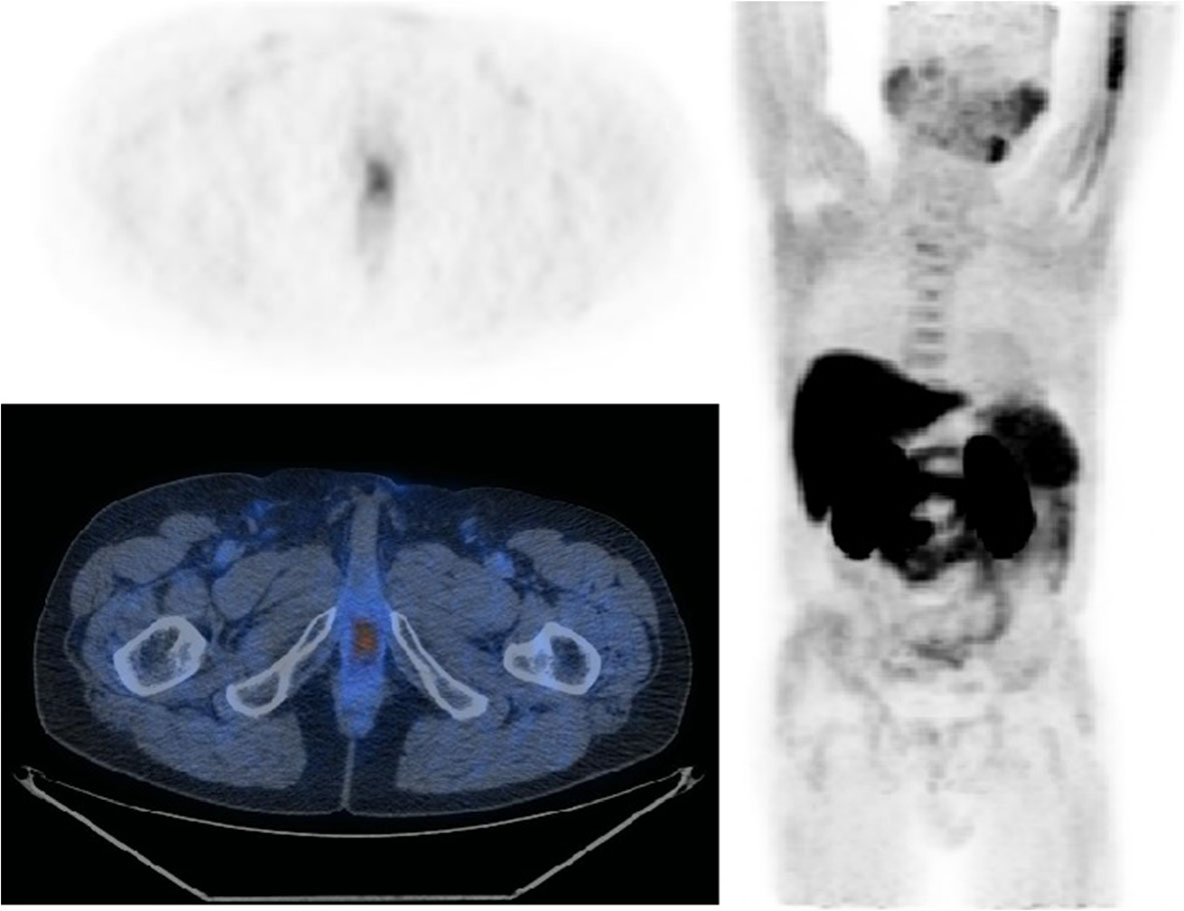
False positive case (PET/CT axial scan). This scan shows a mild focal uptake of 11C–choline localized in the BUJ along midline
